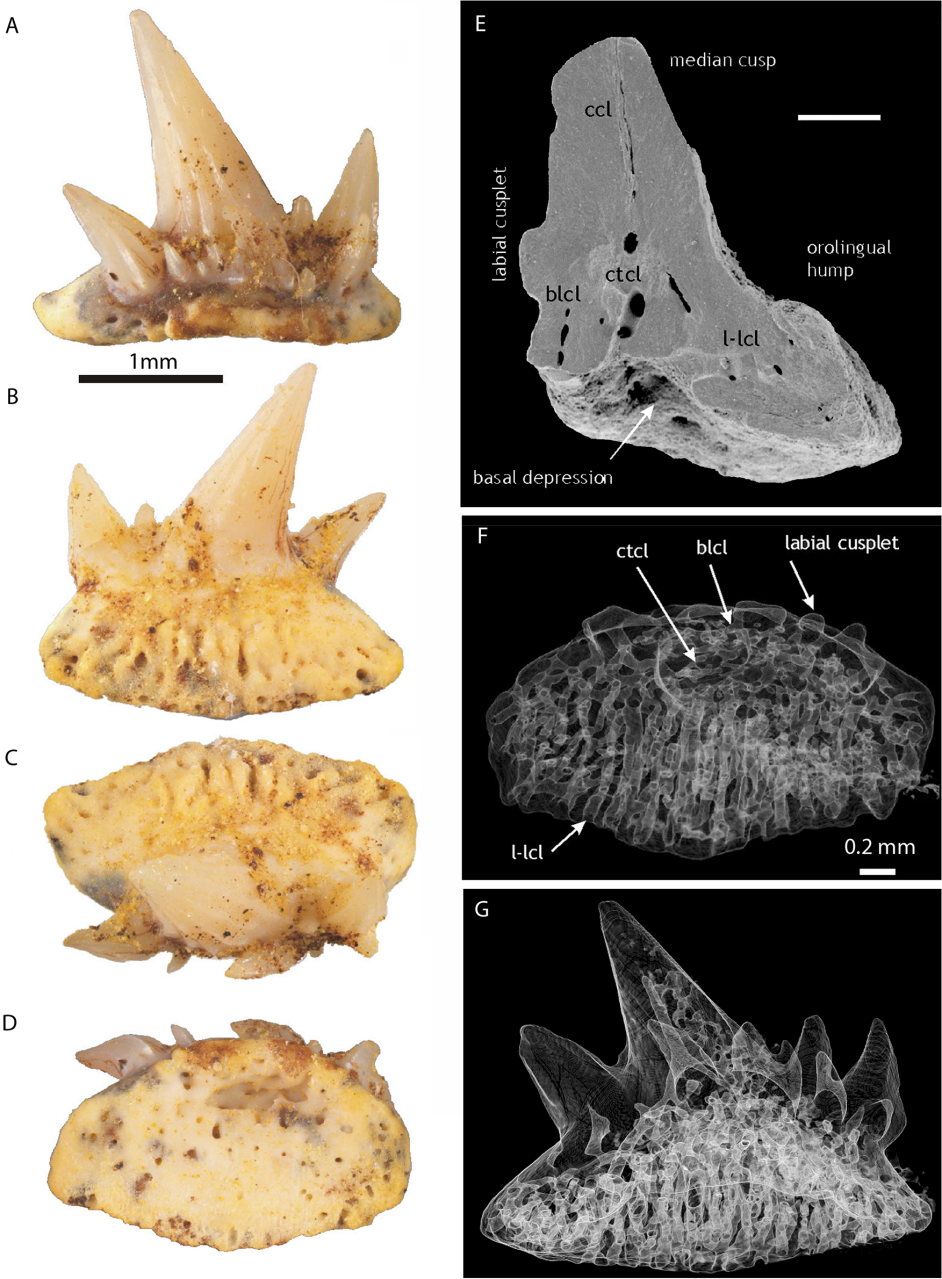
Teeth of *Gogoselachus lynbeazleyae* WAM 09.6.145, Gogo Formation, Western Australia. Large tooth WAM 09.6.145–009 in (A) lingual, (B) labial, (C) oral (D) aboral views, in natural light. (E) SEM of tooth WAM 09.6.145–010, naturally broken near the median surface. (F, G) CT-scans of tooth WAM 09.6.145–011, showing layout of vascular canals, in basal and lingual-lateral views. Abbreviations: l-lcl, labio-lingual canals; ctcl, central canal; blcl,basolabial canals; ccl, coronal canals.
